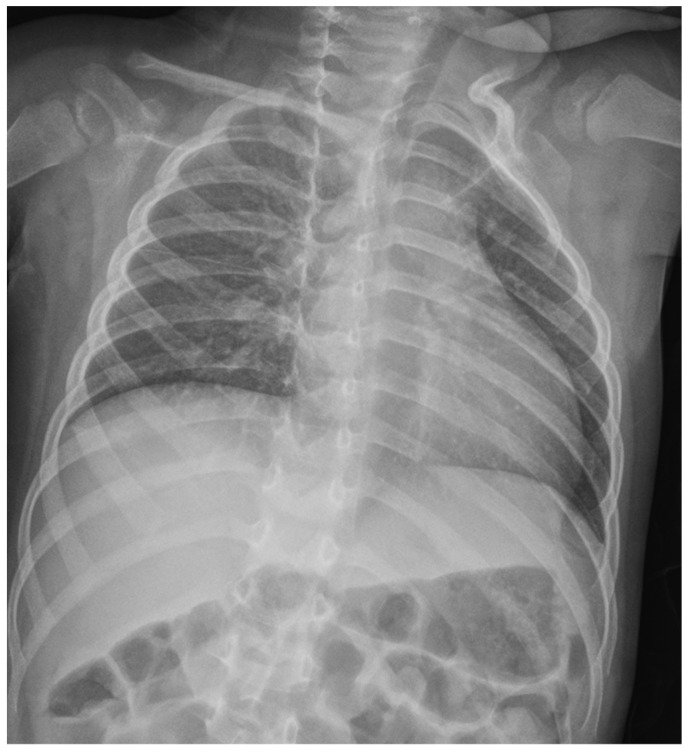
Anteroposterior chest X-ray at admission. The lung fields are clear with normal vascular markings and no evidence of parenchymal consolidation or pleural effusion. The cardiac silhouette is within normal limits for age.
